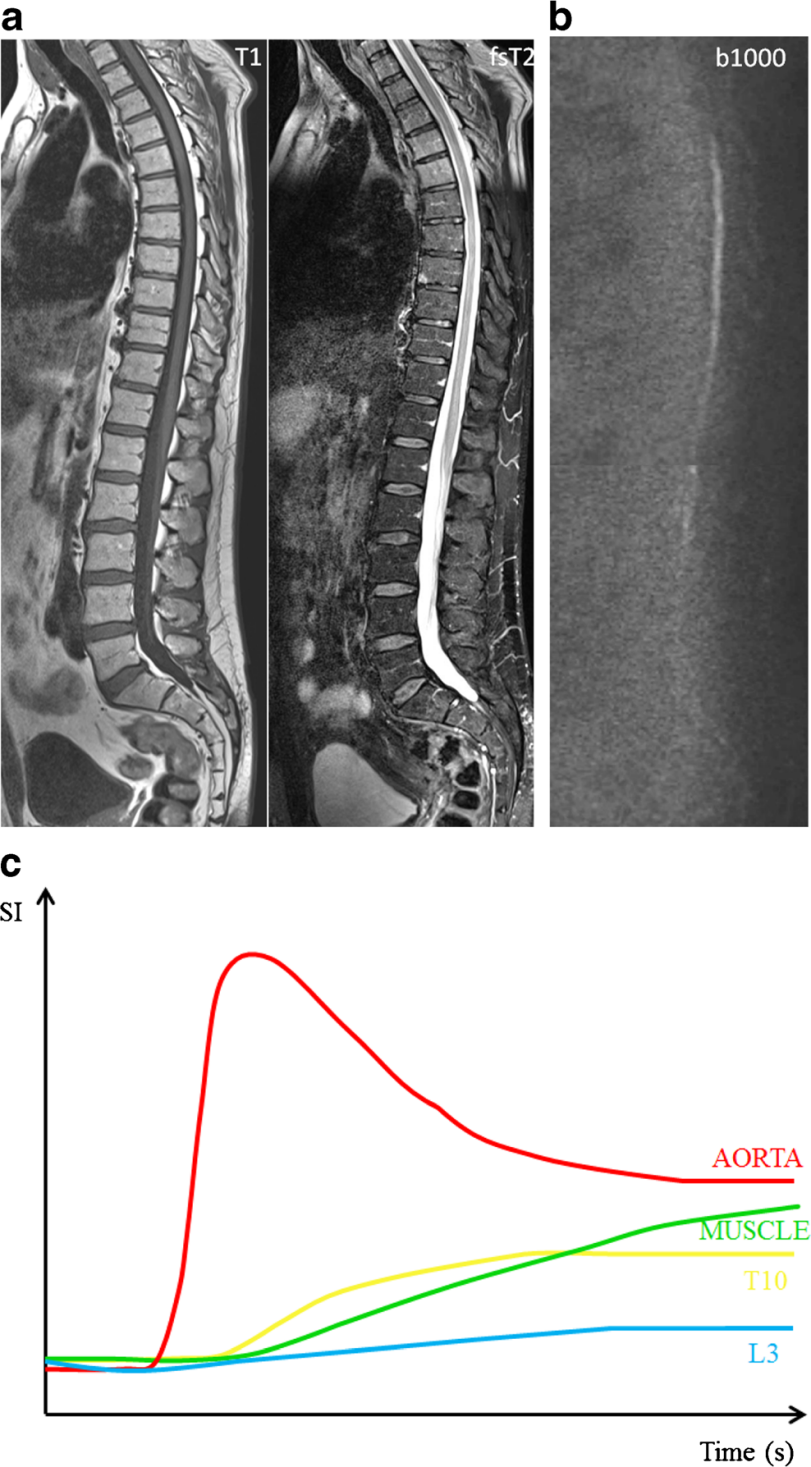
MR images of a patient with monoclonal gammopathy of undetermined significance, with 5 % plasma cells on bone marrow biopsy. a T1-weighted and fat-suppressed T2-weighted images of the thoracolumbar spine displaying normal bone marrow signal intensities, hyperintense on T1 and hypointense on fat-suppressed T2-weighted images. b DWI b1000 images with normal appearing bone marrow, low signal intensity due to the high amount of fat and low cellularity with low water diffusivity. c Time-intensity curve derived from DCE-MR imaging with a TIC type 1 (L3 - *blue*) and type 2 (T10 - *yellow*) curve, corresponding to a normal vascularization of the bone marrow, no signs of neoangiogenesis
